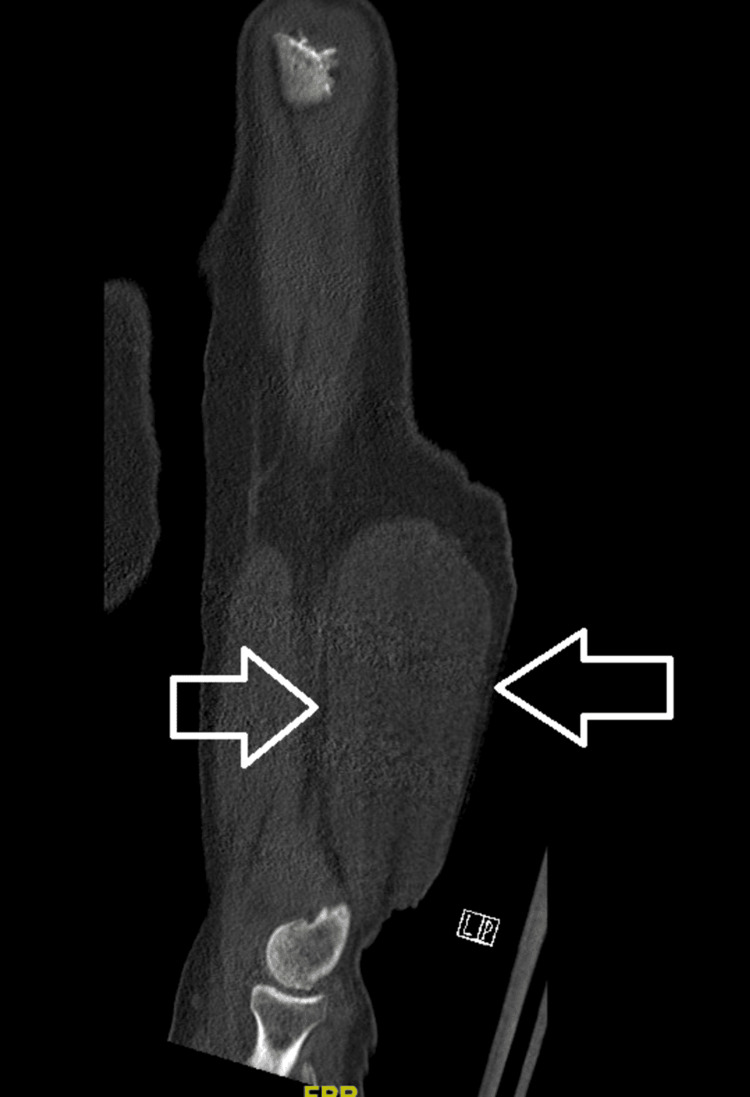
CT scan of the right human humerus The scan shows a 12 x 6.6 x 3.3 cm ovoid fluid-attenuating mass/collection in the posterolateral mid to distal arm located deep to the fascia with mass effect on the triceps. There was no periosteal reaction.
